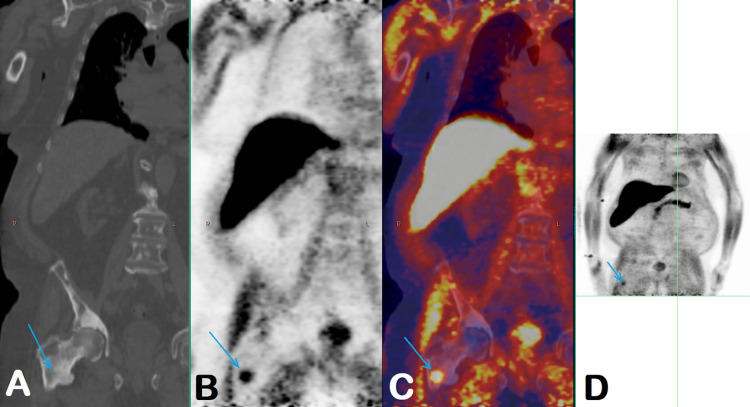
Findings on the fluoride-18 fluciclovine PET-CT scan in October 2021 CT (A), PET (B), fused PET-CT (C), and MIP (D) images show intense focal radiotracer activity in the intertrochanteric region of the right femur (blue arrows), not in the prior sclerotic lesion, but in the adjacent, less dense sclerotic area. It shows SUVmax of 4.1 (for reference, background physiologic bone marrow activity SUVmax of 2.5). PET – positron emission tomography, MIP – maximum intensity projection, SUVmax – maximum standardized uptake value
